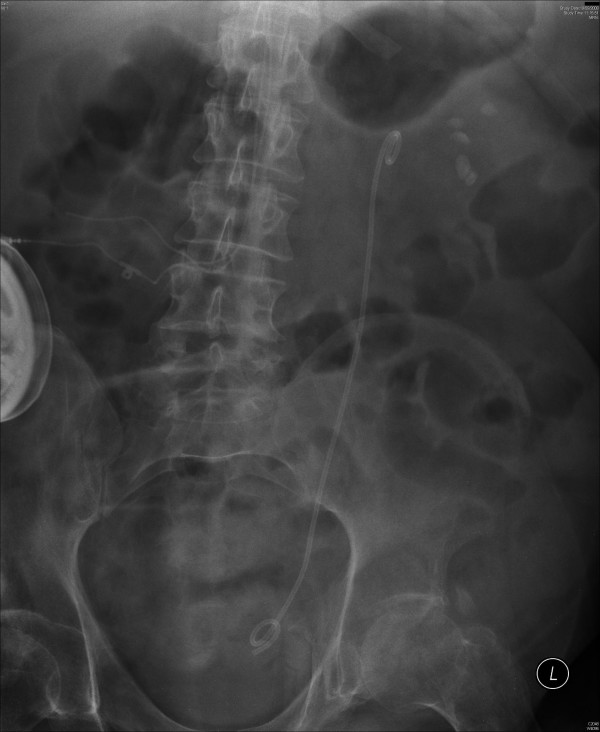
X-ray of abdomen, taken on 19 September 2008, showed significant reduction in the bulk of the previously noted stag horn renal calculus; small residual calculi present in middle and lower pole calyces.
